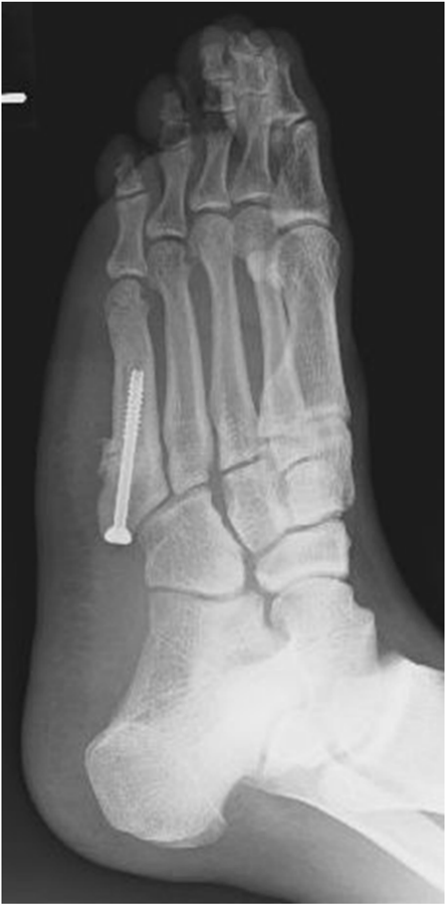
X-ray immediately following surgery. The fracture site was curetted, a cannulated cancellous screw with a diameter of 5 mm was inserted, and autologous bone was grafted.
